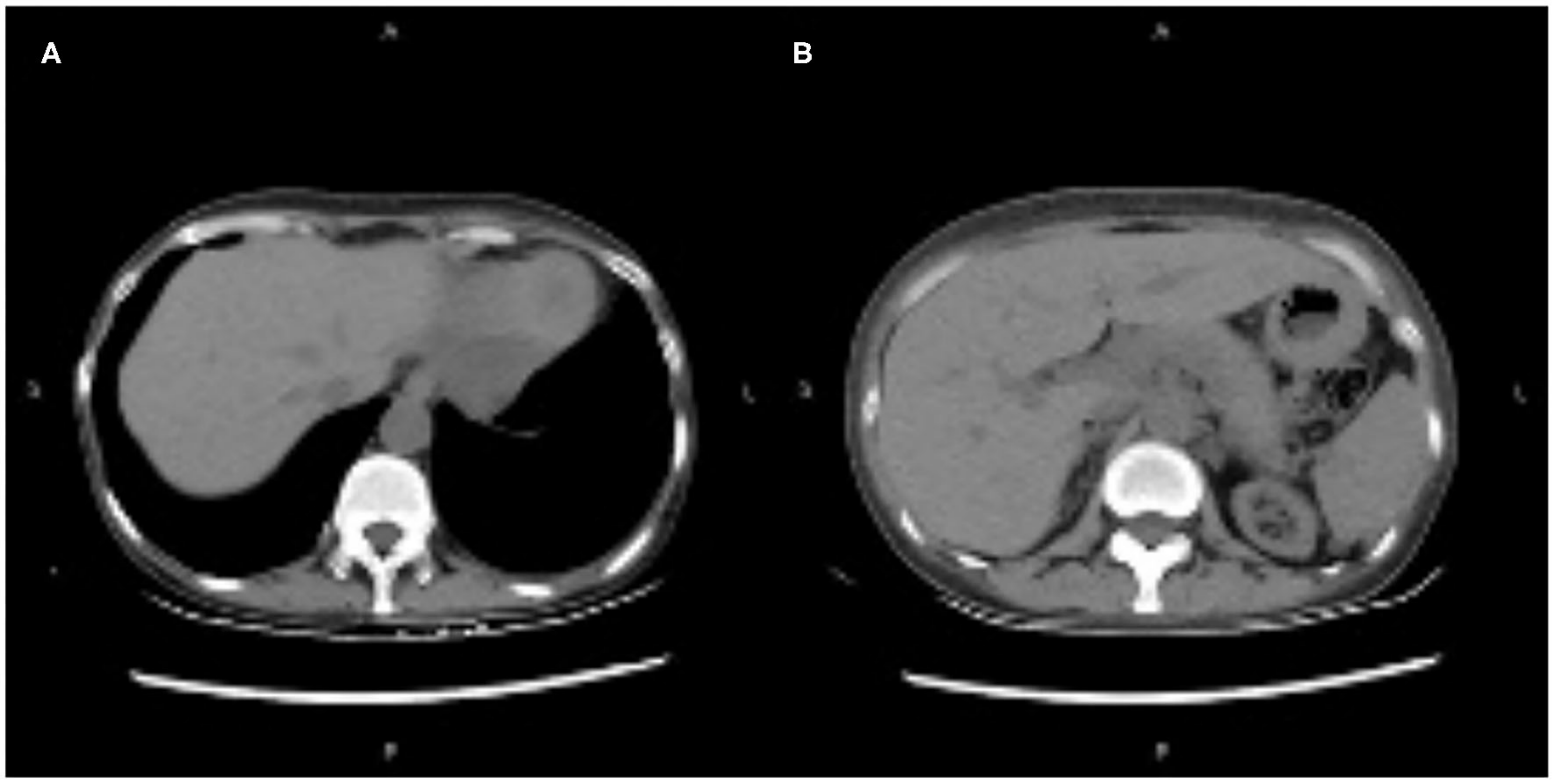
(A,B) Computed tomography showing no effusion in the bilateral pleural cavity, and no exudation around peripancreatic and other areas of the abdominal cavity.
